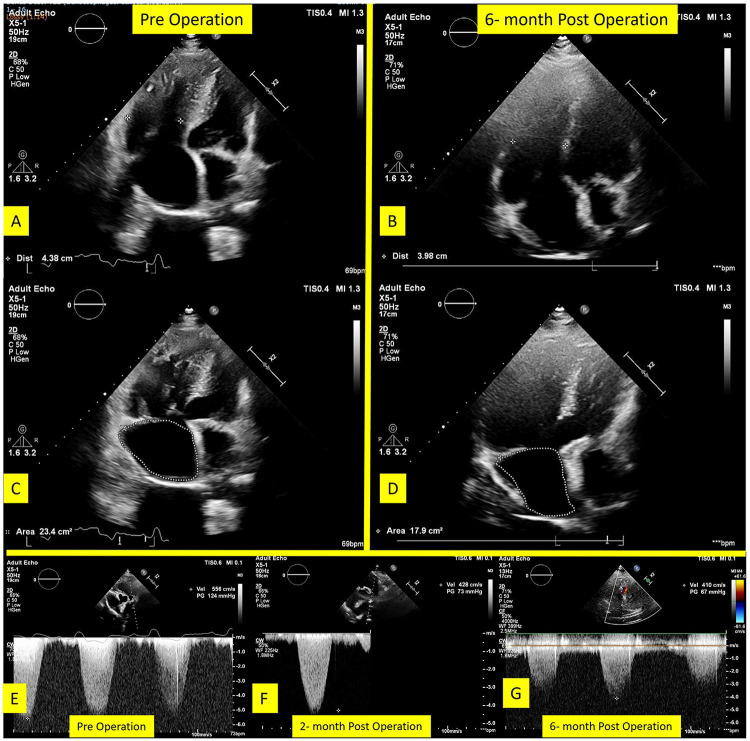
Serial echocardiographic findings before and after surgical repair. Panels (A) and (B) show a reduction in right ventricular diameter from 4.38 cm preoperatively to 3.98 cm at six months. Panels (C) and (D) show a reduction in right atrial area from 23.4 cm² preoperatively to 17.9 cm² at six months. Panels (E–G) show reduction in the RVOT/pulmonary valve gradient from 124 mmHg preoperatively to 73 mmHg at two months and 67 mmHg at six months. These echocardiographic changes suggest postoperative improvement but do not replace CMR-based quantification of right ventricular volumes and function. The residual RVOT gradient remained significant and requires ongoing ACHD follow-up.
